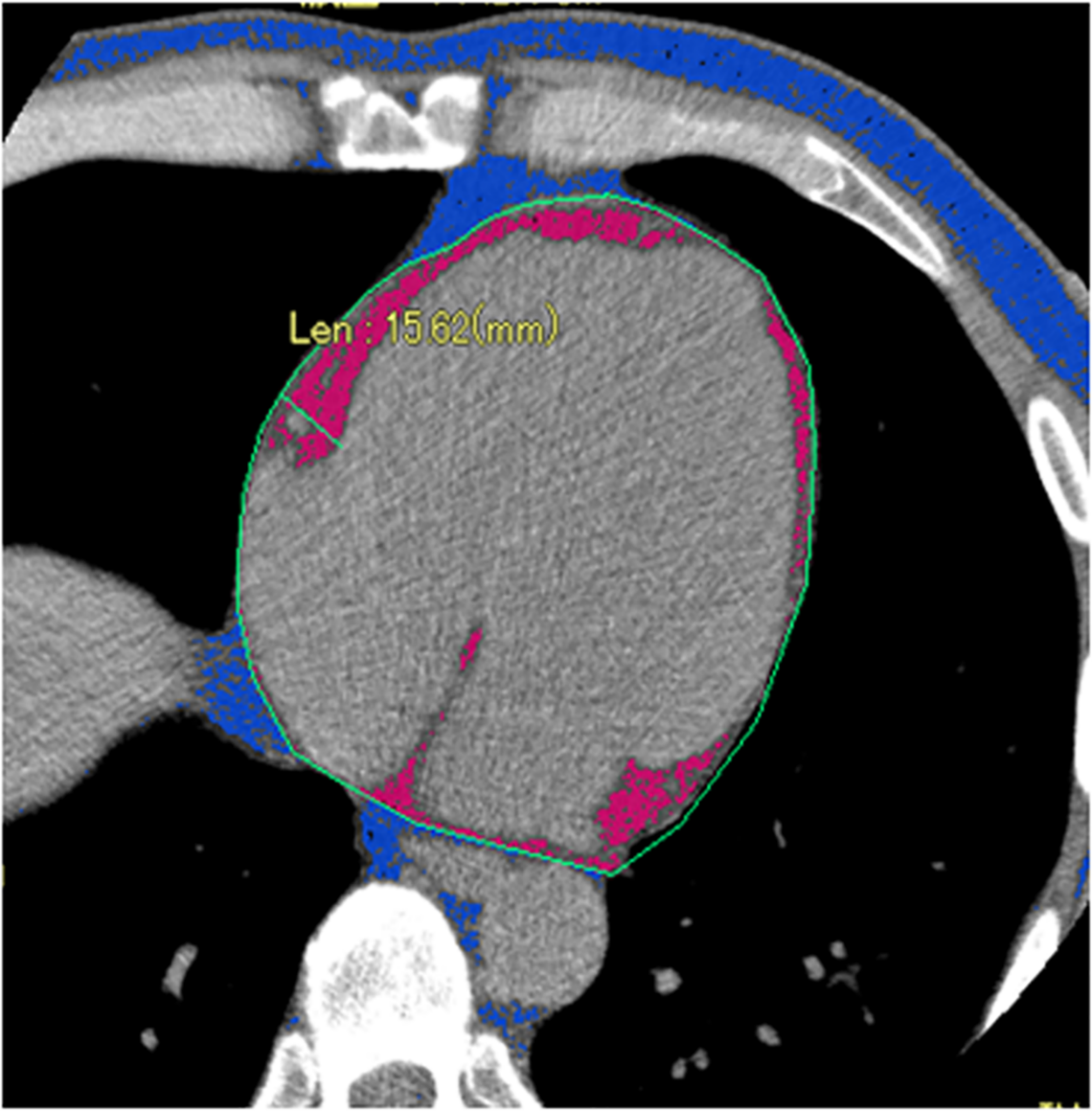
The representative image of CT image evaluating EAT. A region of interest (ROI) was manually traced along the visceral pericardium as indicated green line. A density range between -200 and -30 Hounsfield Units was used to isolate adipose tissue displayed as pink area.
